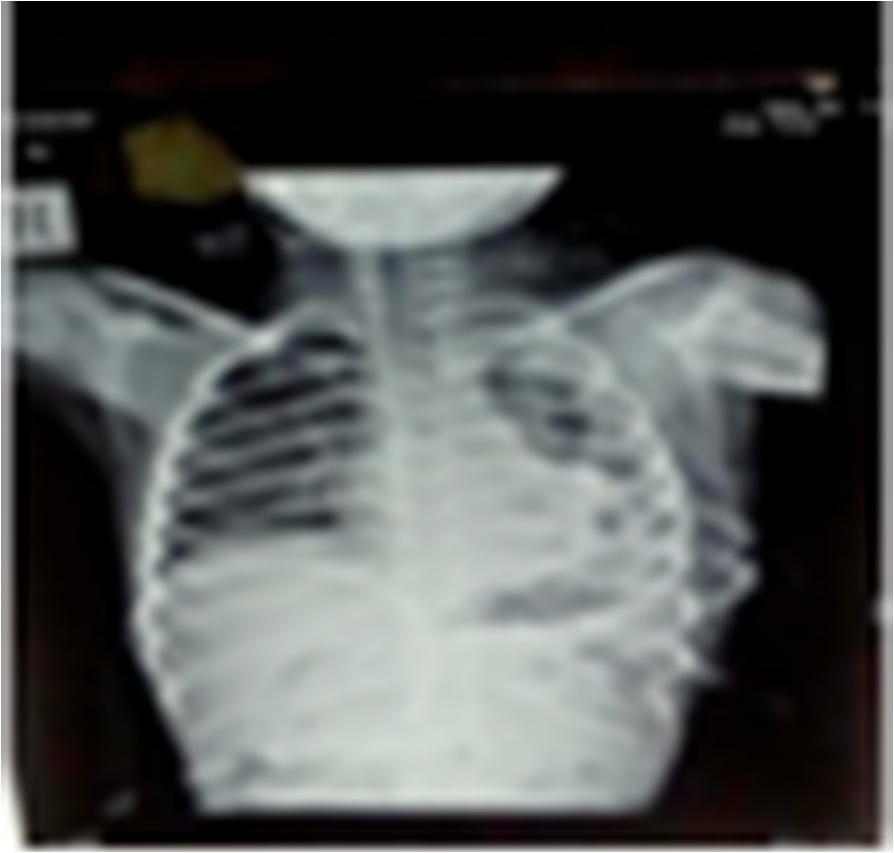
Post-operative X-Ray- showing left lower lobe lobectomy status with chest tube in situ.
